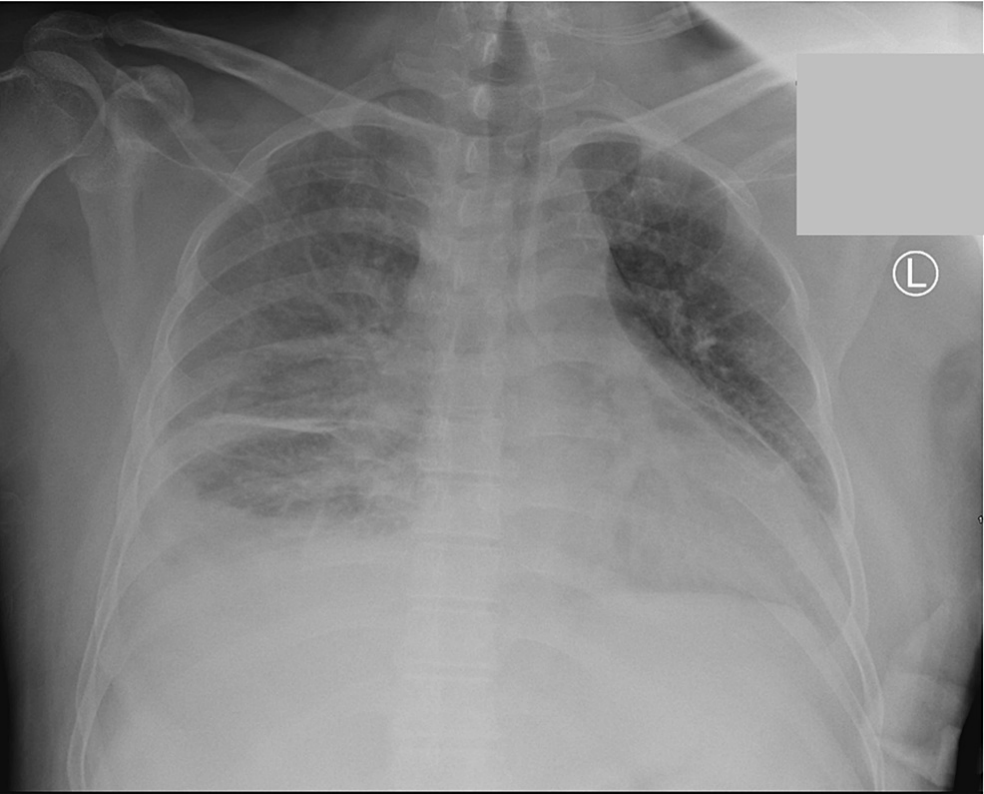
Chest X-ray showing bilateral interstitial infiltrates and right-sided pleural effusion
